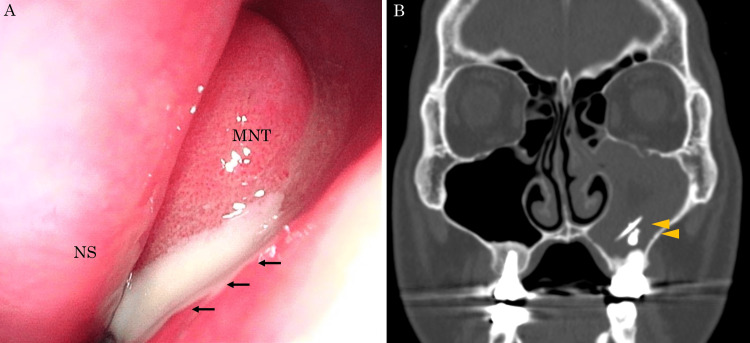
Nasal endoscopy and sinus CT at first visit (A) Endoscopic image of the left nasal cavity. Purulent discharge is observed (arrows). (B) Sinus CT shows a soft tissue density lesion filling the left maxillary sinus with a rod-like and spherical radiopaque mass (arrowheads). CT: computed tomography; MNT: middle nasal turbinate; NS: nasal septum
